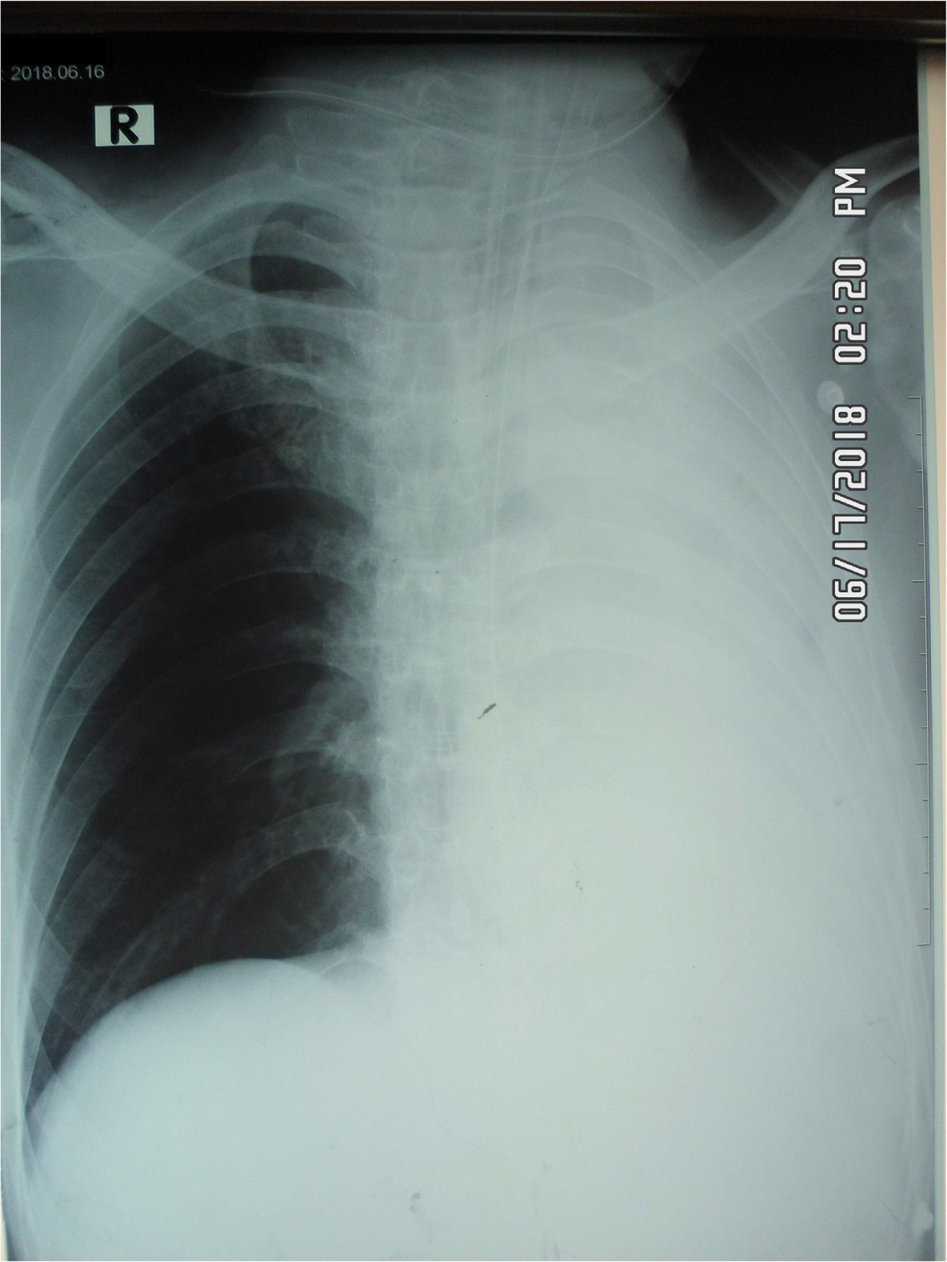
Chest X-ray anteroposterior view showing complete left lung collapse and hyperinflated right lung with right-sided subcutaneous emphysema
